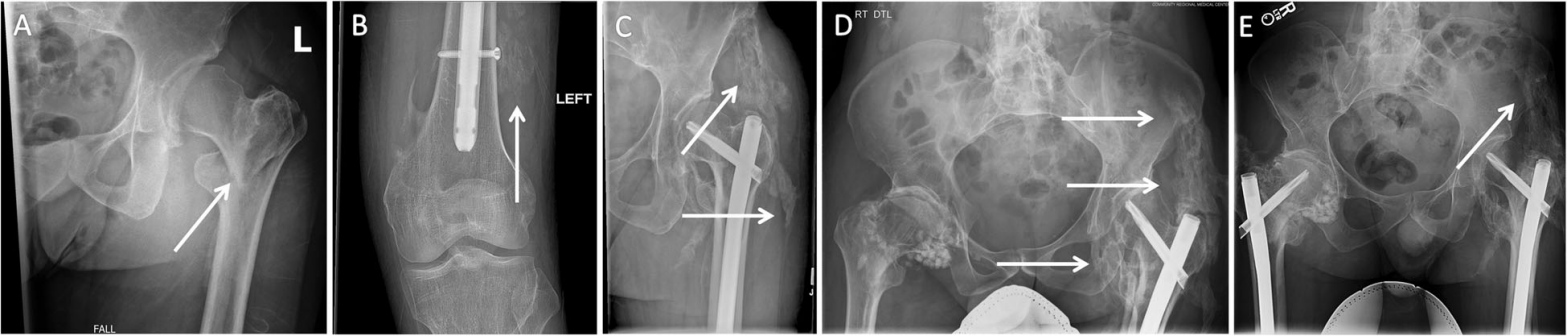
X-Ray imaging of the left hip: (a) X-Ray of the left pelvis at the time of the subtrochanteric fracture [arrow]. (b) X-Ray of the knee 4 months after surgery with HO at the site of distal screw insertion [arrow]. (c) Brooker class D HO at the rod insertion site 4 months after surgery [arrows] and after 9 months (d). (e) X-ray showing matured HO formation at 12 months [arrow]
